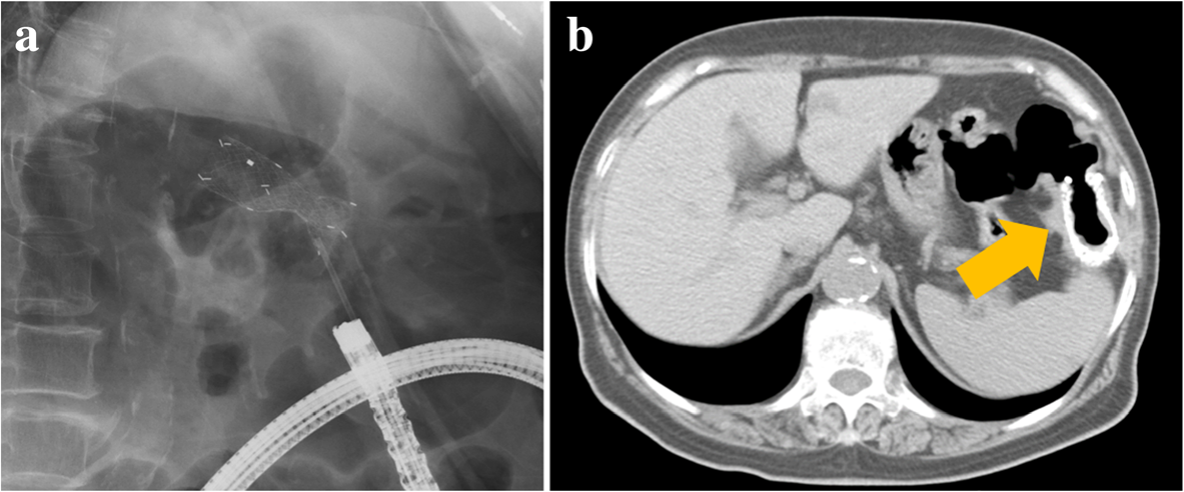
Fluoroscopy and computed tomography findings after colonic stent placement. a Colonic stent was placed in the descending colon under fluoroscopic guidance. b Colonic stent (yellow arrow) reduced the obstruction
